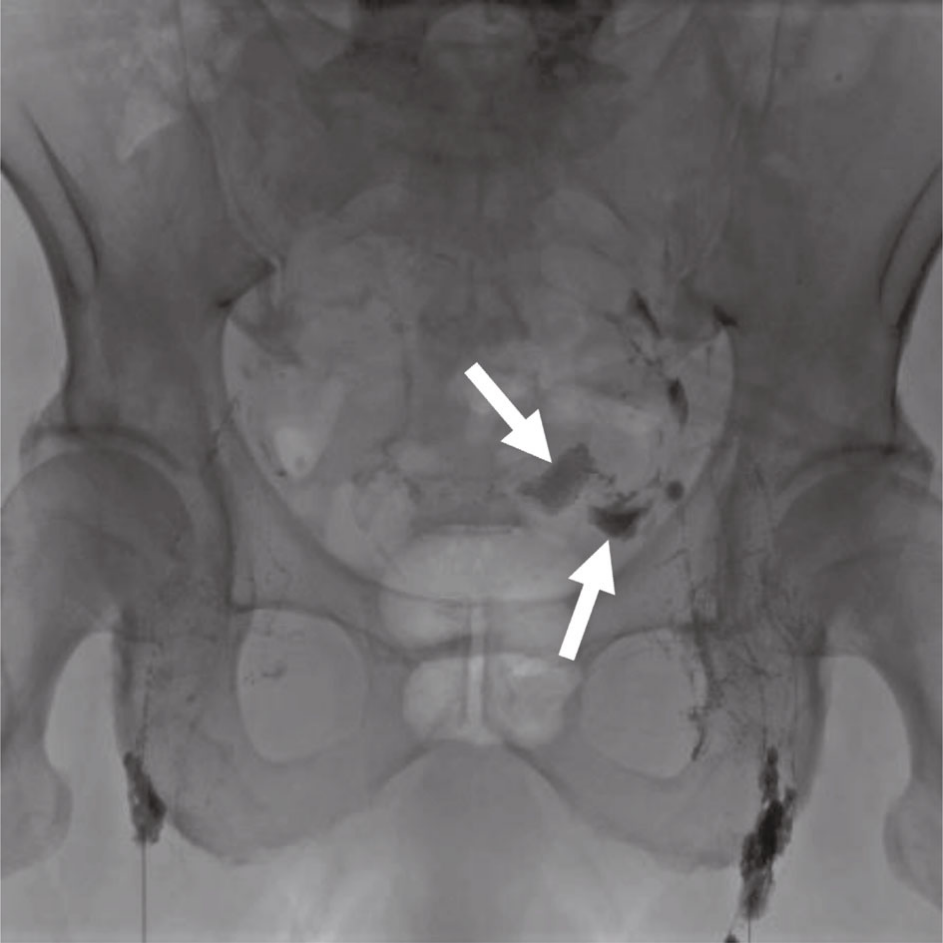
Pelvic X-ray performed during intranodal lymphangiography demonstrating leakage of lipiodol (arrows) from the left wall of the pelvis into the peritoneal cavity.
